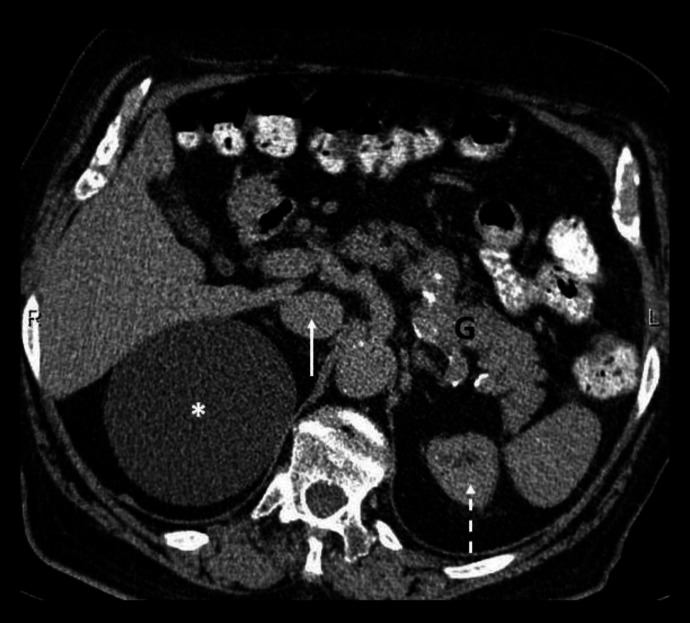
Contrast enhanced axial CT abdomen of a 56- year-old male shows a well-defined, homogenous, non-enhancing, low density lesion (asterisk) that on inferior images was found to be arising from the upper pole of the right kidney. The findings are suggestive of an exophytic simple renal cyst. A simple cyst, irrespective of its origin, is characterized by absence of calcification, septation, heterogeneity, nodularity, wall thickening or enhancement on post contrast images. Solid and dashed white arrows point to IVC and upper pole of the left kidney respectively. Specks of calcification seen close to pancreas (G) involve the tortuous splenic artery
